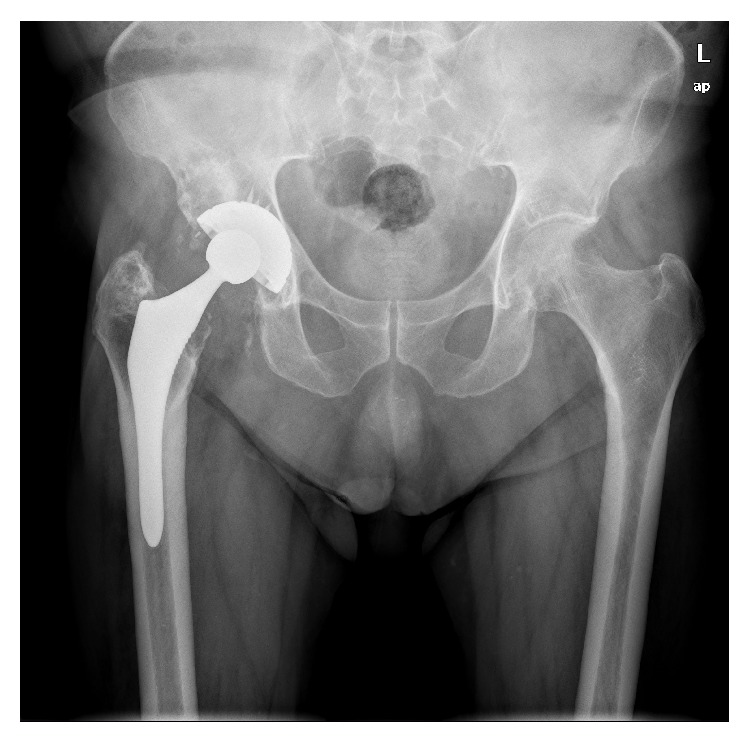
Same case as in Figures 2, 3, and 4. X-ray control image in 2013, after 8 y. follow-up of second surgery with original implant since 1993.
